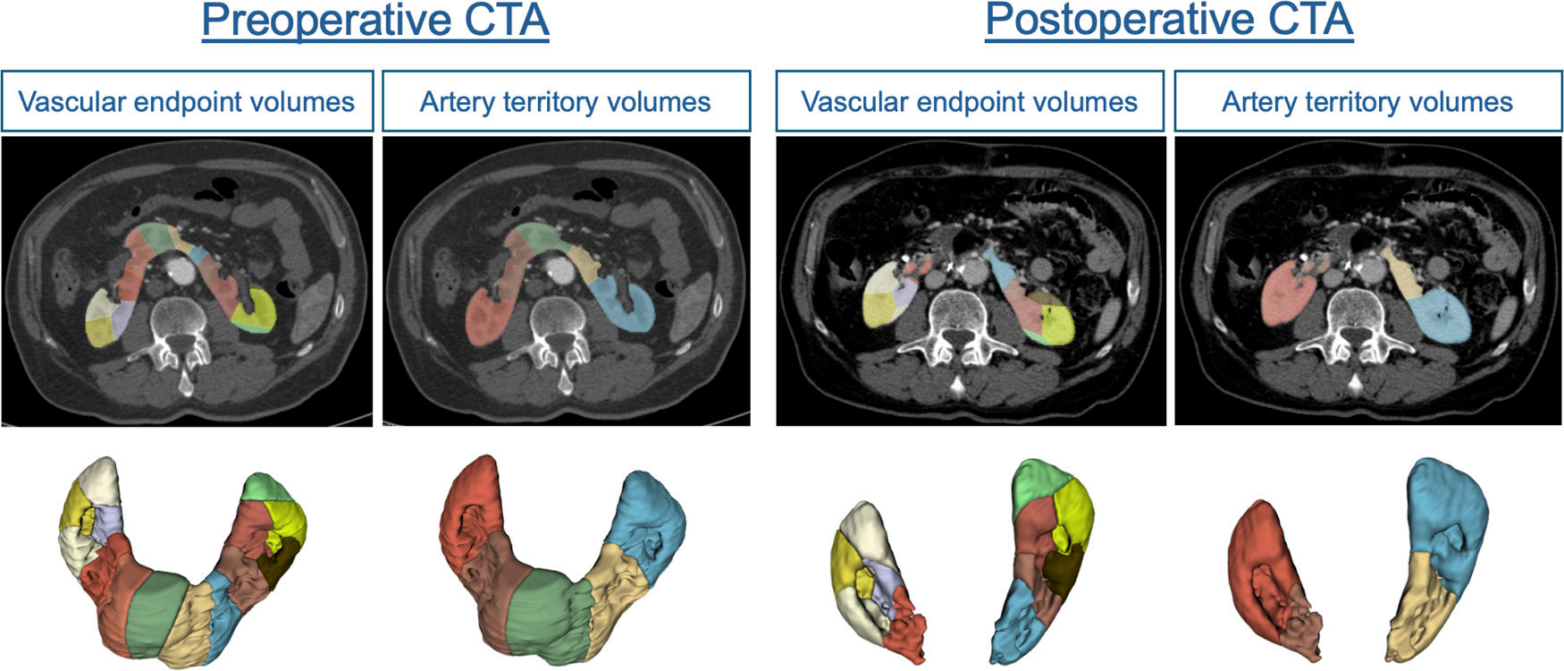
Visual results of the obtained segmentation for both preoperative and postoperative CTA in patient 3. CTA, Computed tomography angiography
